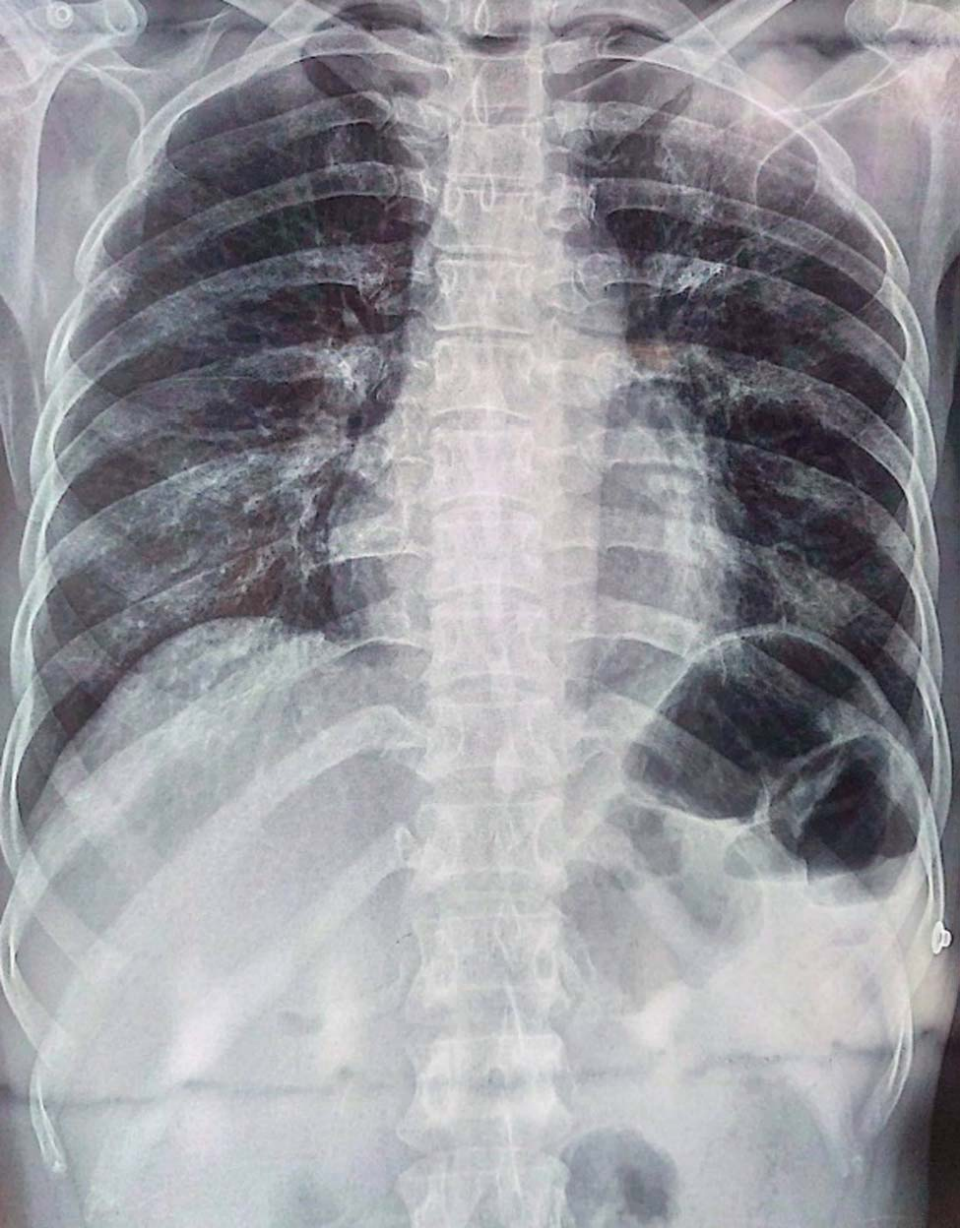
Chest radiograph did not show any distinct abnormal features.
